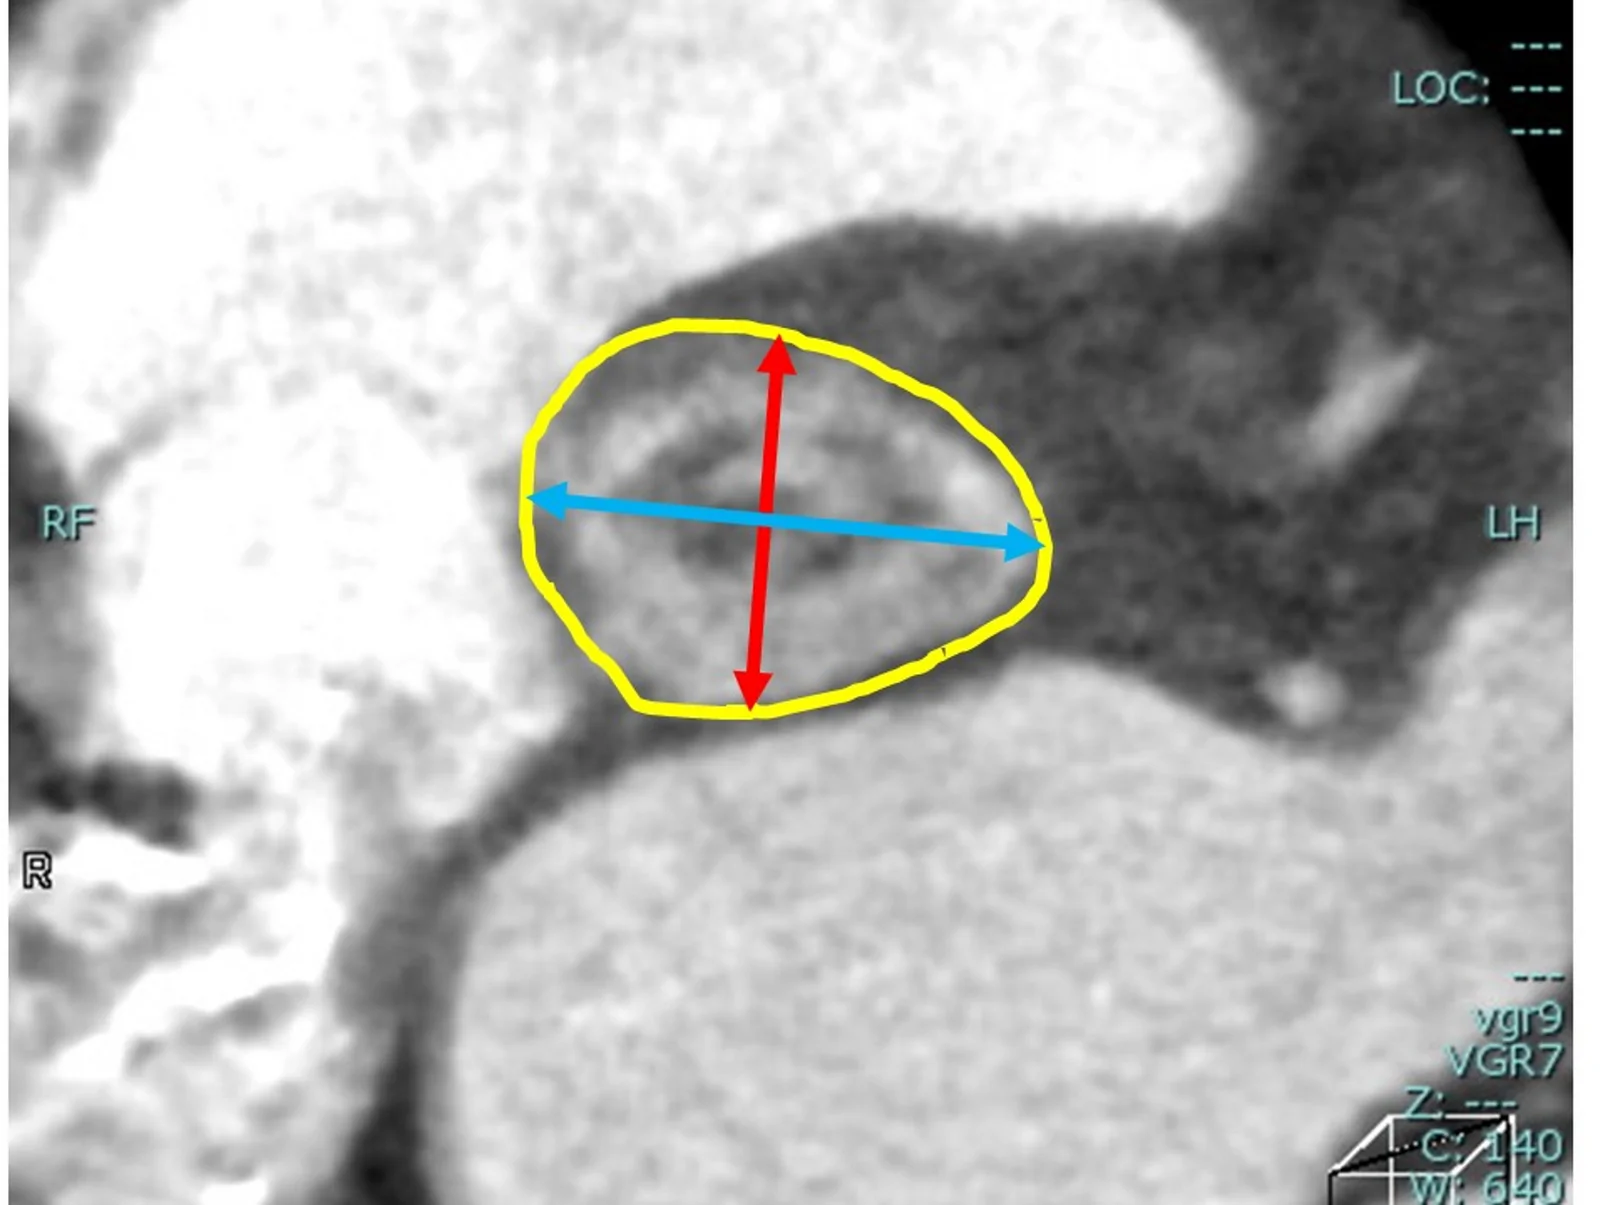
Preoperative computed tomography before the initial aortic valve replacement. Measurement of the left ventricular outflow tract (LVOT) before the initial surgery for infective endocarditis. The LVOT is outlined by the yellow line. The maximum diameter is indicated by the red line, and the minimum diameter is indicated by the blue line. The LVOT circumference was 83.2 mm, with a maximum diameter of 30.9 mm and a minimum diameter of 21.7 mm, corresponding to an area of 5.18 cm^2^. A low-attenuation lesion within the LVOT is suggestive of vegetation
